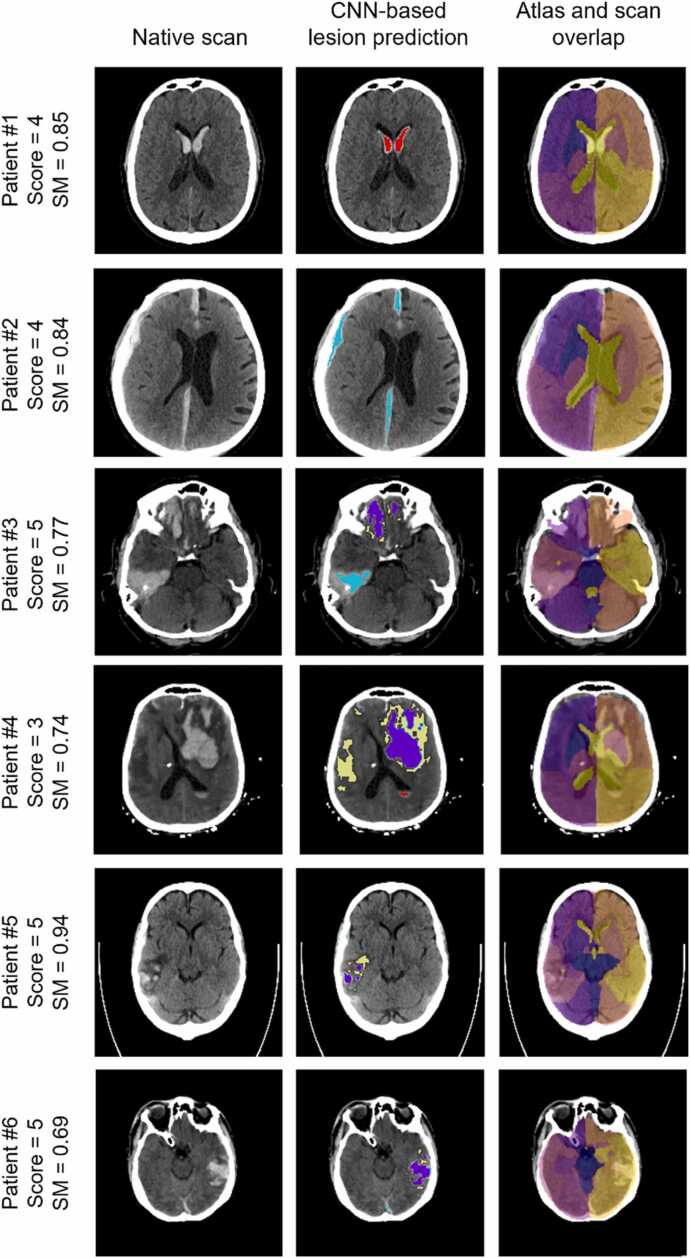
Qualitative atlas mapping results from Dataset 2, with corresponding manual score and SM value. Images in neurological orientation. Lesion map prediction (from BLAST-CT) colour legend: Red - IVH; Purple - IPH; Yellow - Oedema; Light blue – EAH.
